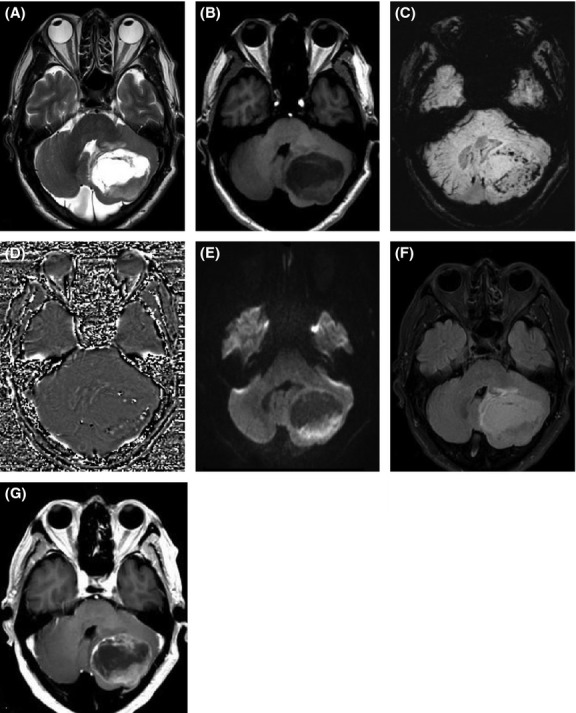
A 60-year-old female with metastatic neuroendocrine carcinoma (the primary sites of cancer was lung). One lesion was located in left cerebellar hemisphere on T2WI (A) and T1WI (B). There were no vessels and microhemorrhage shown on T1WI and T2WI. However, on SWI-MinIP (C), multiple hypointense signals regarded as hemorrhage and vessels were found in the lesion, which showed hyperintense signal on phase image (D). The lesion showed hyperintense signals both on DWI (E) and FLAIR (F), and demonstrated solid, intense and inhomogeneous enhancement on contrast-enhanced T1-weighted image (G).
